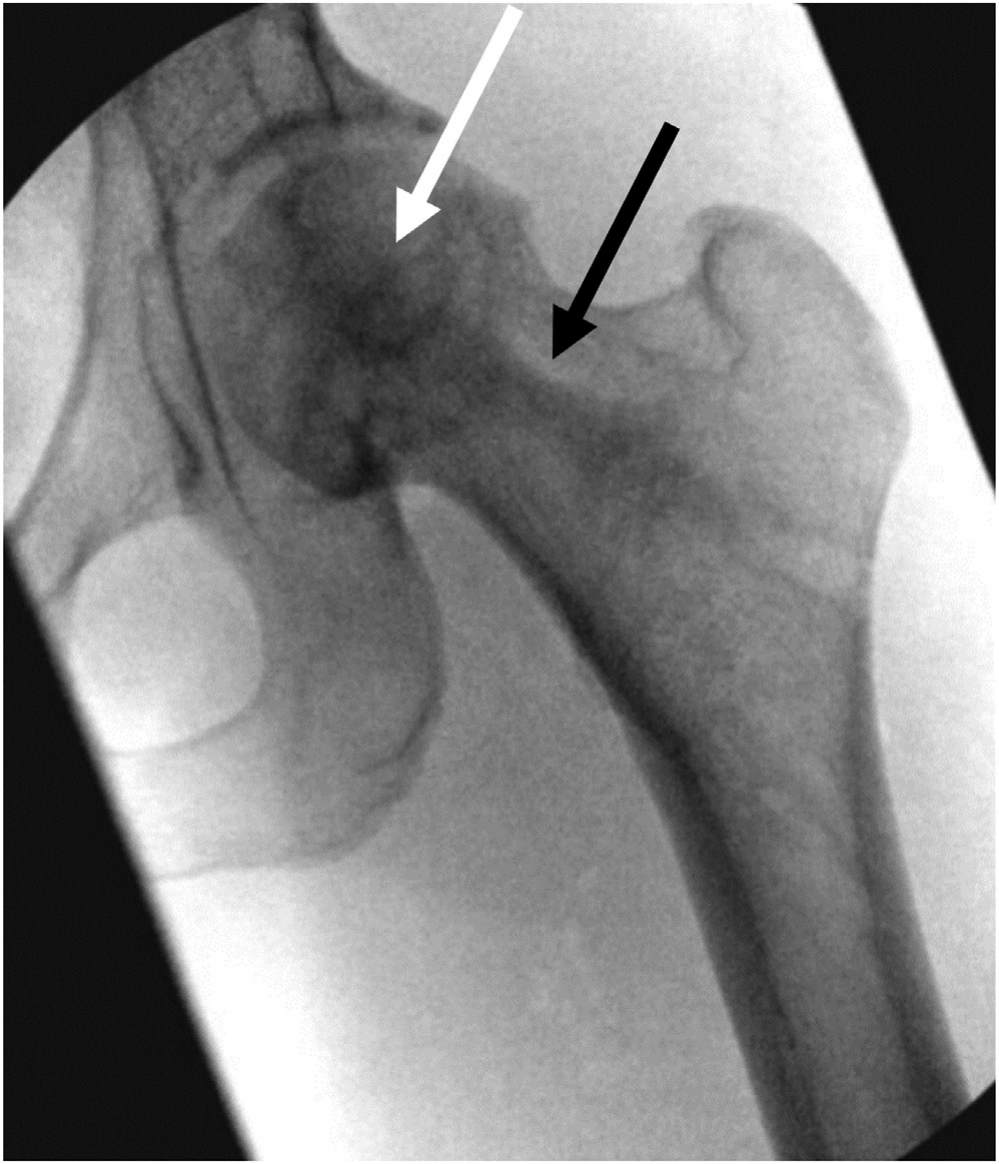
Final radiographs of the left hip demonstrating completed core decompression and bone grafting. Graft is placed in the decompressed area of the femoral head (white arrow) as well as along the reamer path (black arrow).
